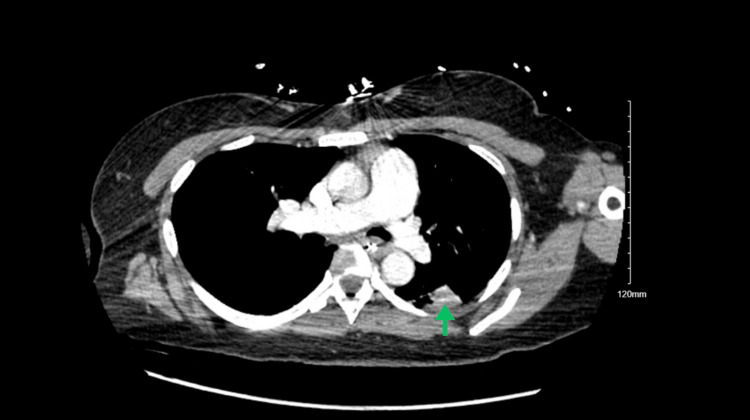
Computed tomography (CT) scan with contrast demonstrating a left basilar pulmonary arteriovenous malformation measuring up to 2 cm. Green arrow: Left basilar pulmonary arteriovenous malformation.
